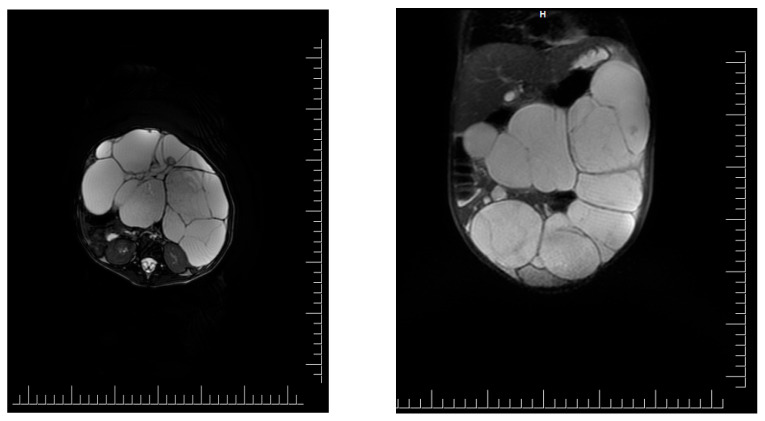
MRI scan of intrabdominal mass. Cystic masses in close relation to intrabdominal structures are observed. However, on an M.R.I. scan, no involvement of the intrabdominal structures is observed.
